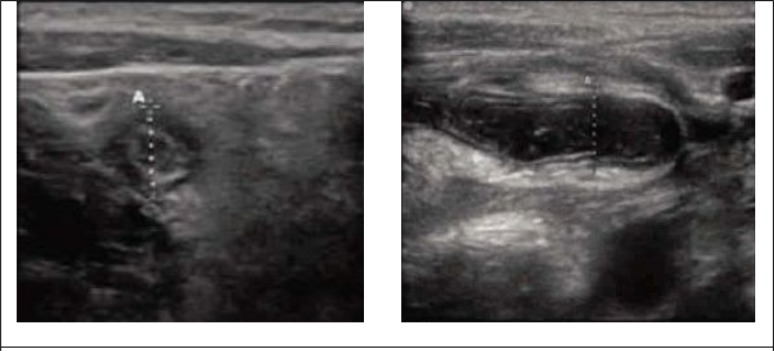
Sonographic views of appendix.
